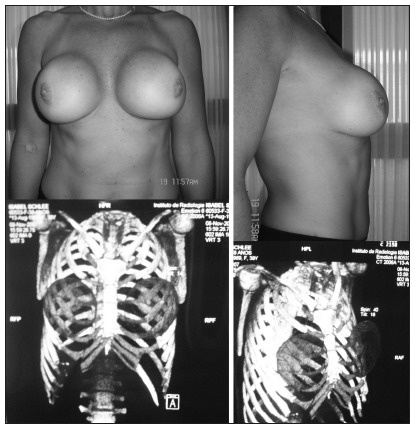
Photographs of the patient and multi-slice helical computed tomography two years after the operation.
